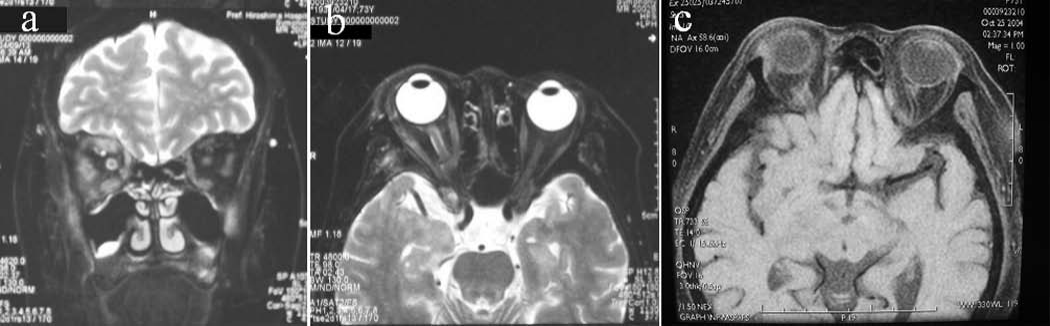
Fat-suppressed T2-weighted MR images of Case 1. Coronal image (a) and axial image (b) from a 73-year-old Japanese woman with idiopathic optic perineuritis. There is a high signal intensity area around the right optic nerve and moderate swelling of the right extraocular muscles, suggesting inflammation around the optic nerve sheath and the extraocular muscles. Fat-suppressed T1-weighted magnetic resonance image obtained one month after steroid pulse therapy. This axial image (c) shows persistence of the high signal intensity area around the right optic nerve and moderate swelling of the right extraocular muscles. The extraocular muscles showed persistent moderate swelling (not visible on this image).
